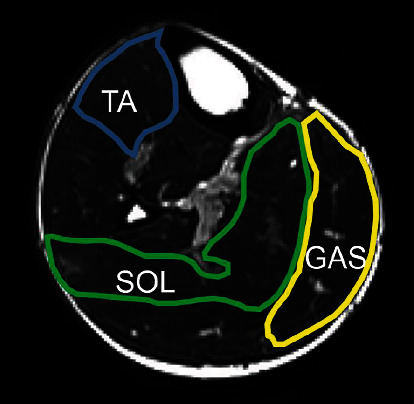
Methods for measuring the ROIs. The ROIs of the tibialis anterior muscle (blue square), soleus muscle (green square), and medial head of gastrocnemius muscle (yellow square) on a T2-weighted image. TA: tibialis anterior muscle; SOL: soleus muscle; GAS: gastrocnemius muscle.
